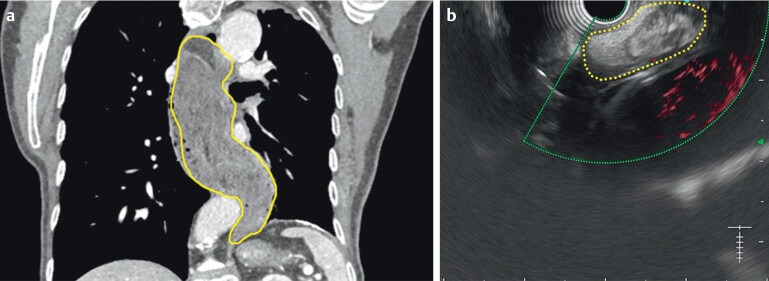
Preoperative computed tomography (CT) and endoscopic
